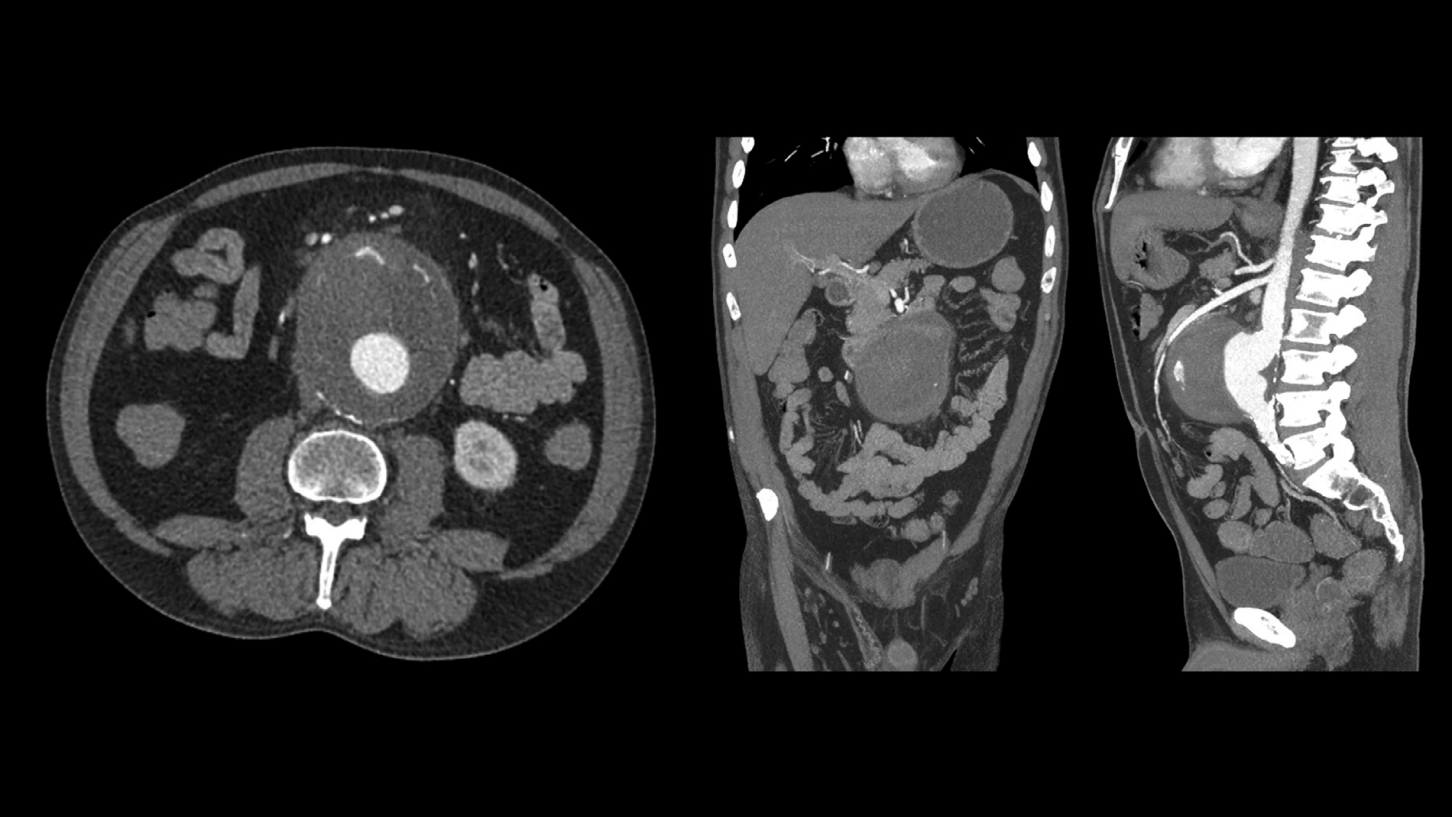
CT abdomen and pelvis with contrast was performed which showed infrarenal abdominal aortic aneurysm measuring 8.9 × 10.3 cm.
